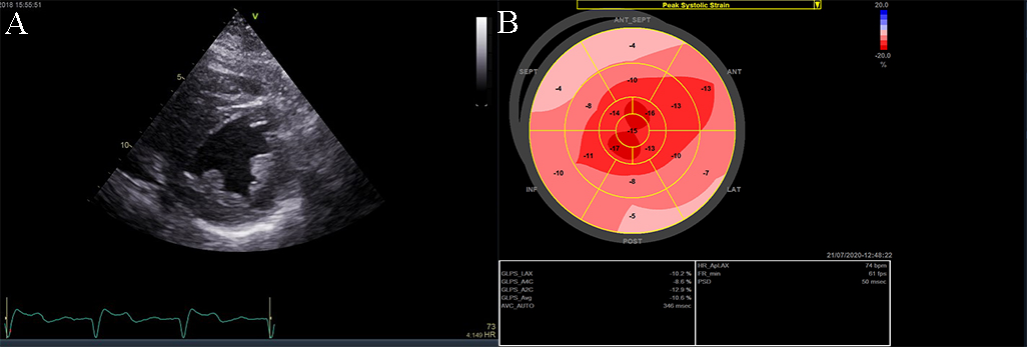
The echocardiographic aspect of cardiac amyloidosis, associated
